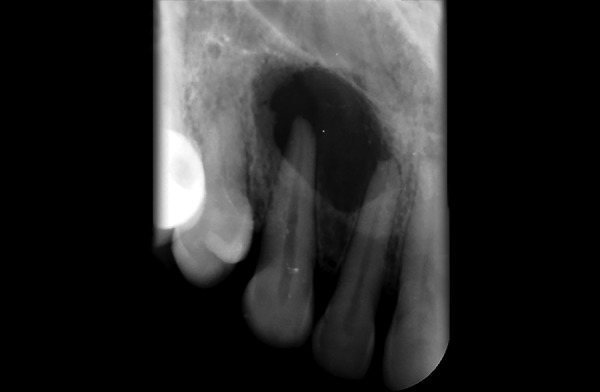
Intraoral periapical w.r.t. 12,13 showing periapical radiolucency
